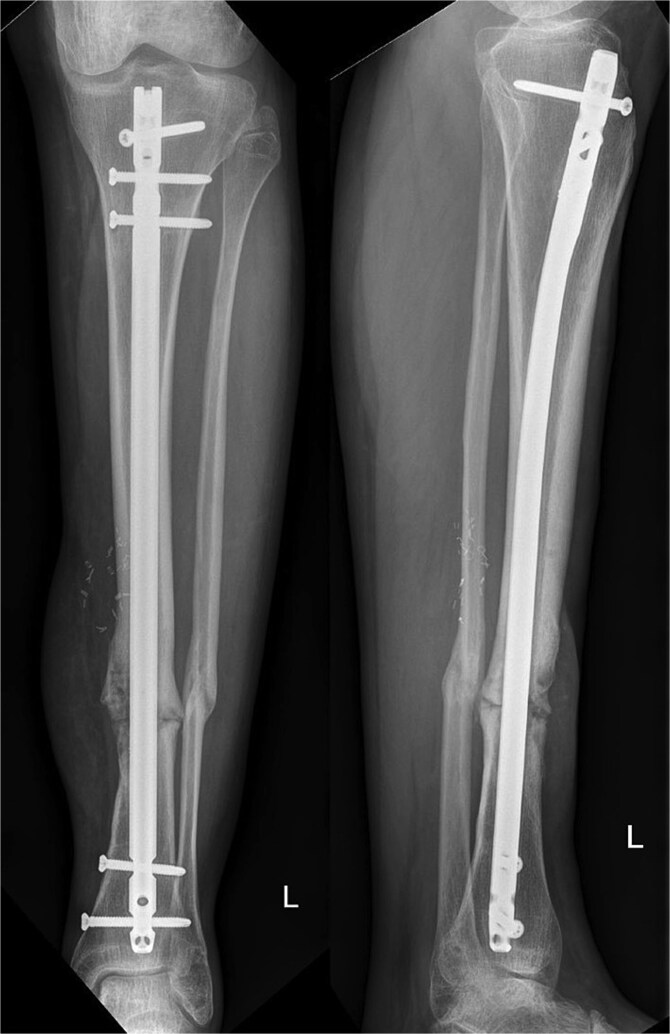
X-ray of lower extremity after 10 months.
